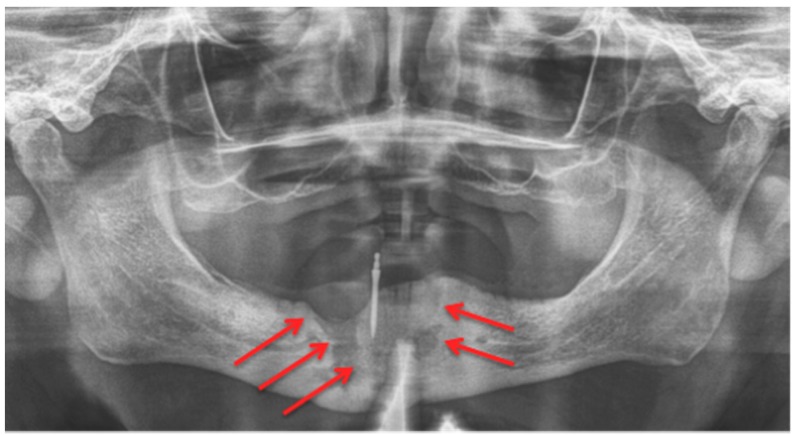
Panoramic radiograph: Patient: 77 years old, male, metastatic prostate cancer, ibandronic acid & later another antiresorptive drug: denosumab. Red arrows point to the necrotic area. Artefact due to thyroid shield. For the corresponding cone-beam computed tomography (CBCT) image, see Figure 4. AAMOS staging: stage 2.
